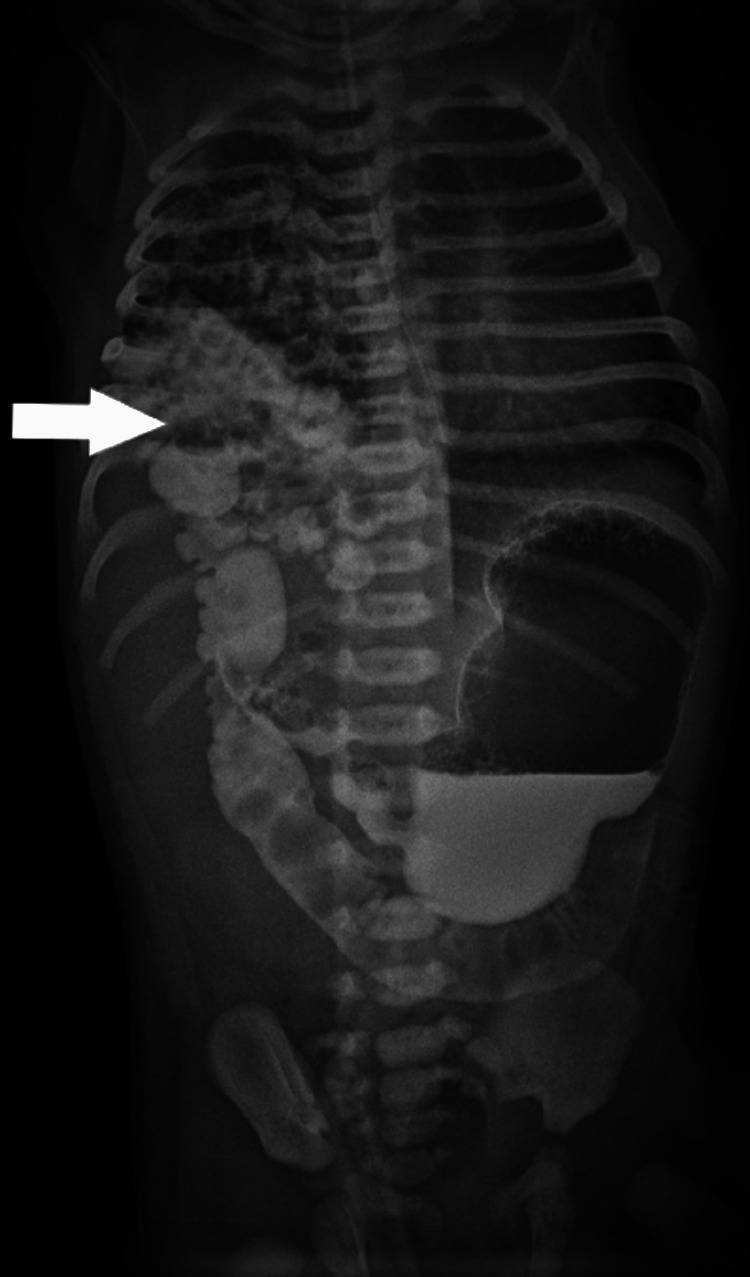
X-ray showing protrusion of bowel in the right hemithorax (arrow) along with malrotation of the gut.
